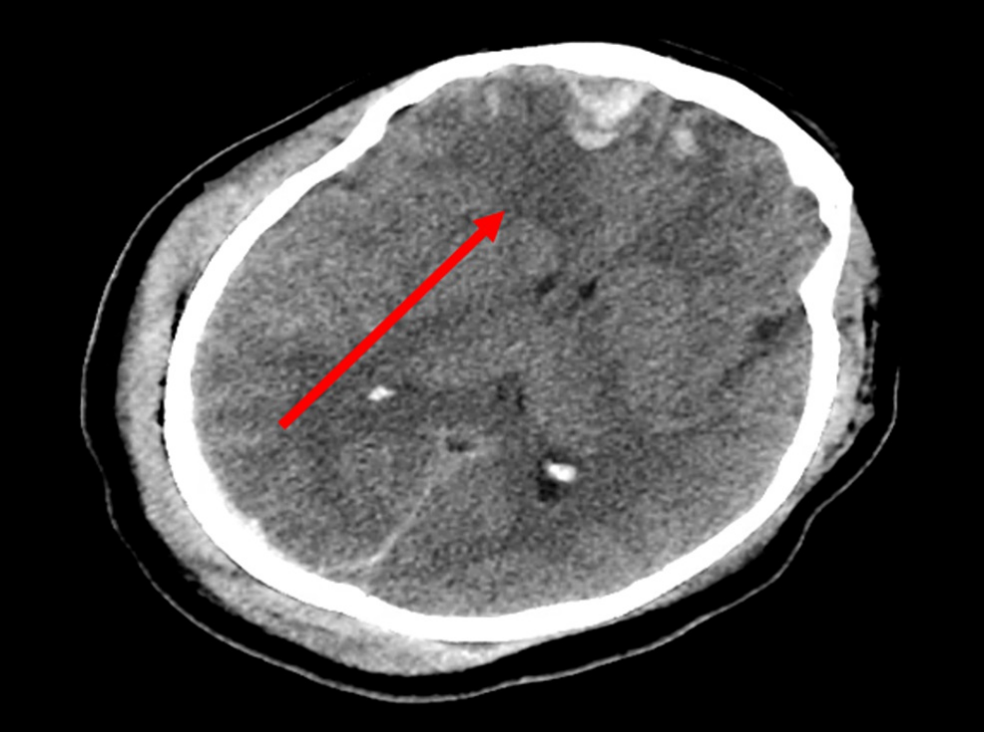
Brain CT six hours after initial presentation showing the progression of intracranial hemorrhages (arrow)
